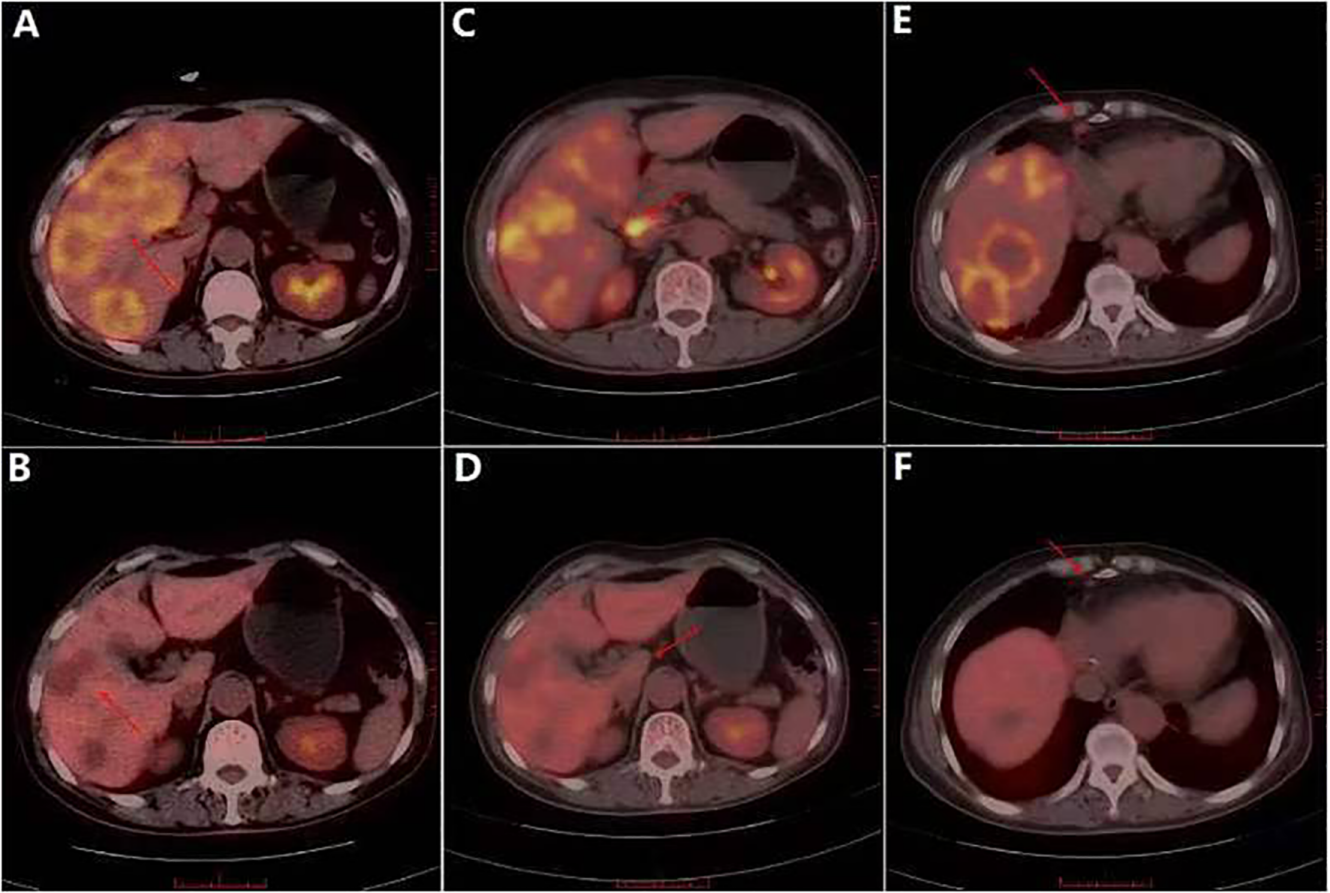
In August 2013, PET/CT showed liver and multiple lymph node metastases. (A) PET/CT showed multiple patchy and flaky low-density shadows of different sizes in the liver. (B) PET/CT showed the disappearance of patchy low-density shadows in the liver. (C) Multiple lymph node enlargement and increased glucose metabolism in right septal angle. (D) No swelling or increased glucose metabolism was observed in the right septal horn lymph node. (E) Local glucose metabolism of the sacrum was increased. (F) Local glucose metabolism of the sacrum was reduced.
